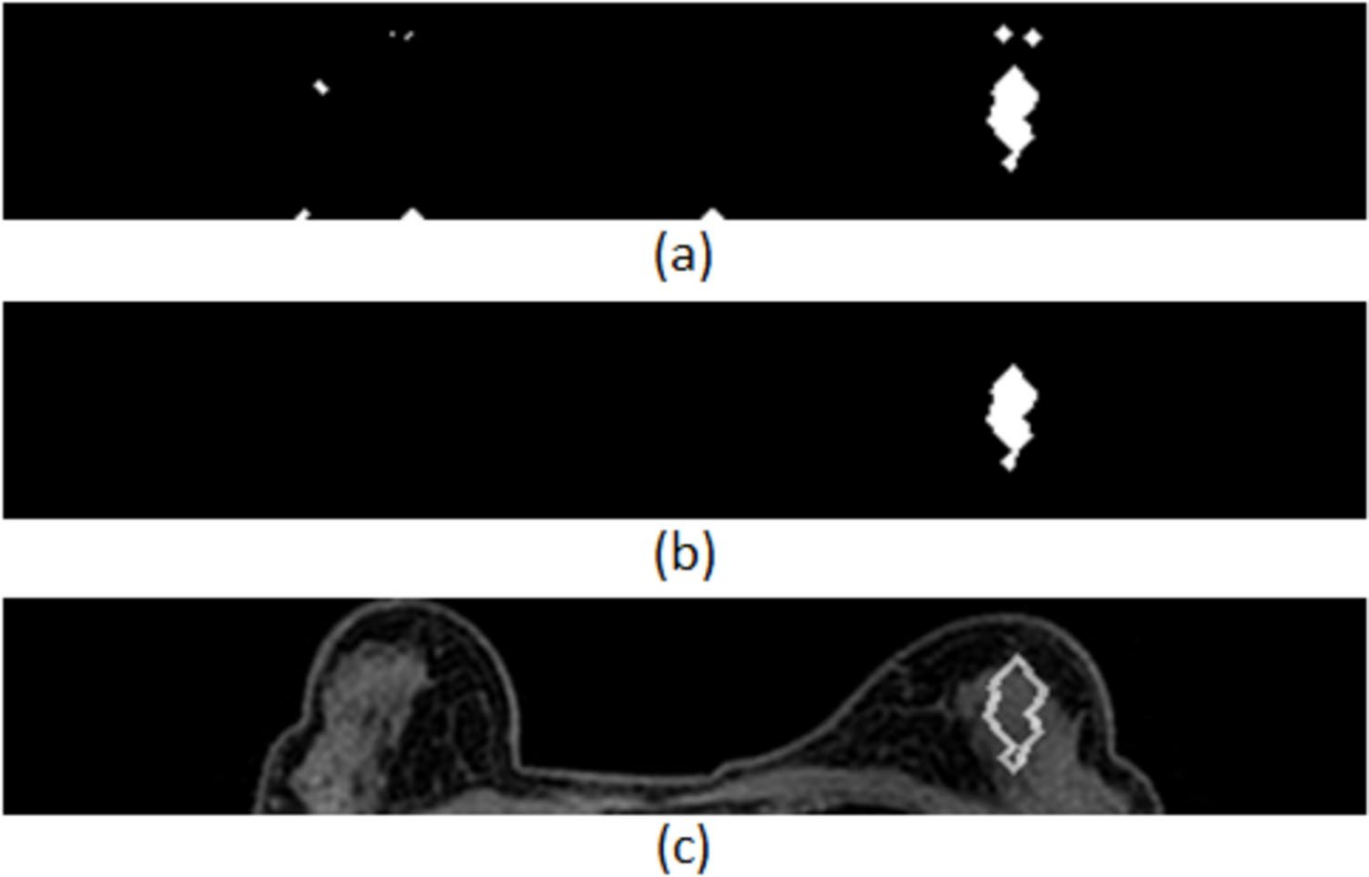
Suspicious areas reduction: a initially extracted areas, b suspicious areas remained after the application of the first classifier, and c outline of the remaining areas overlaid on the original MRI slice
